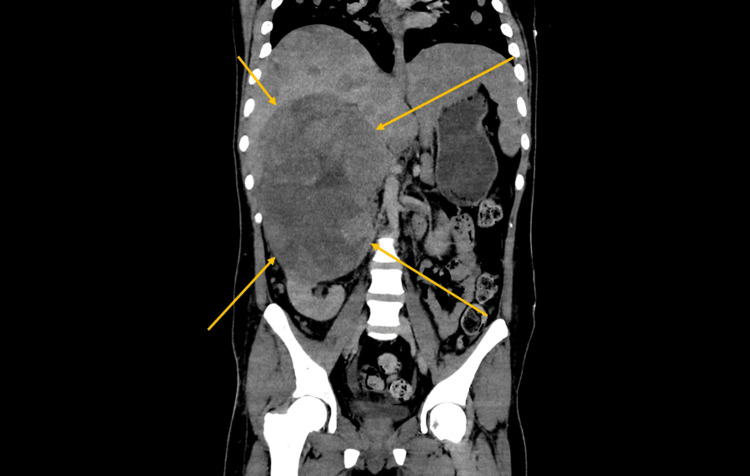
CT scan revealed progression in both size and metastatic spread of the adrenocortical cancer.
